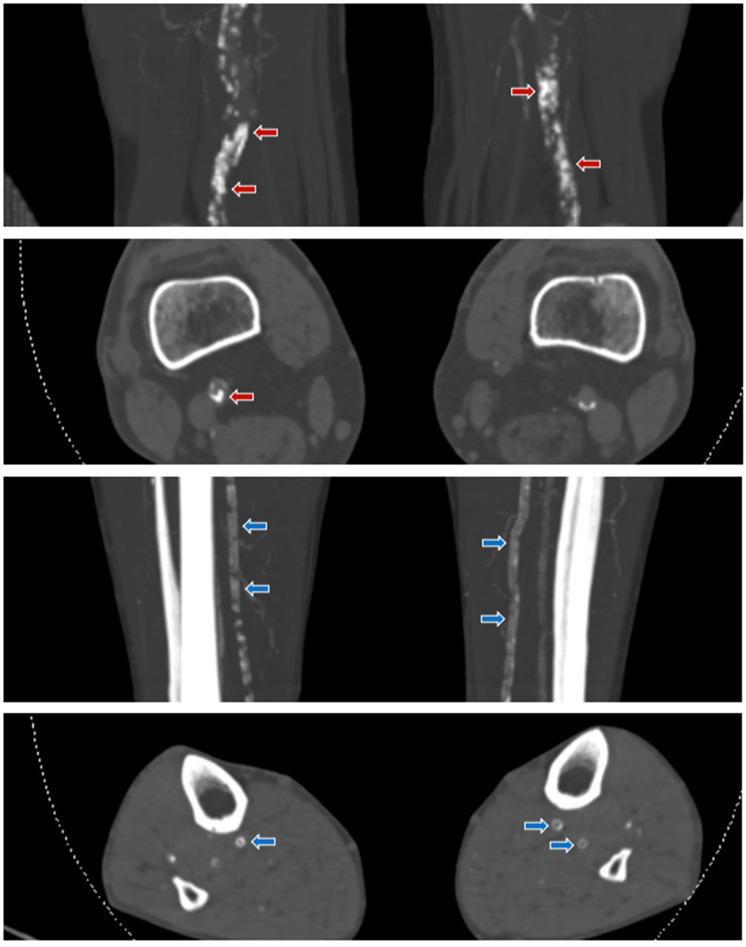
Examples of different calcification patterns in patients with CLI. Shown are coronal MIP and axial 3 mm CT angiography images of the lower extremities. A. Top two figures: the red arrows in femoropopliteal arteries showing irregular/patchy, thick, and non-annular calcifications corresponding to a dominant intimal calcification pattern. B. Bottom two figures: the blue arrows in the crural arteries showing continuous, thin, and annular calcifications corresponding to a dominant medial calcification pattern.
